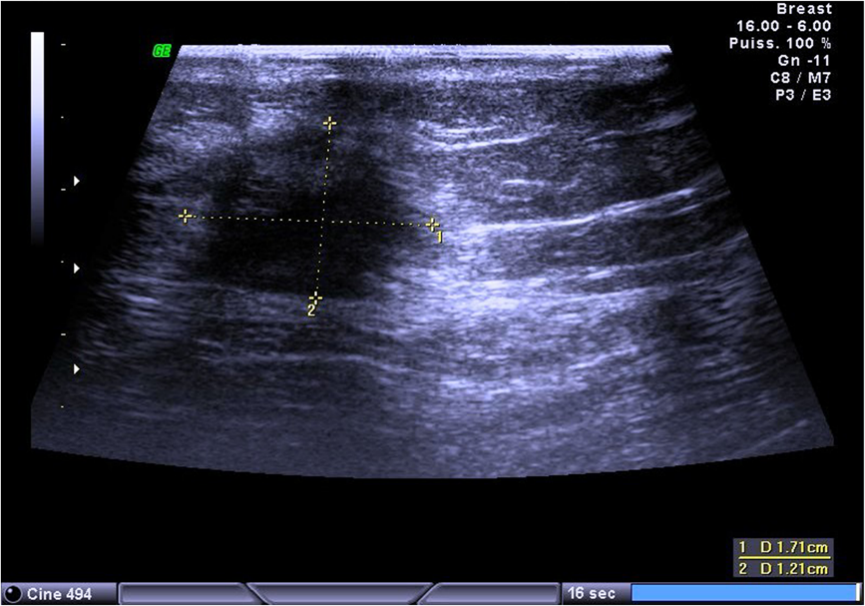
Ultrasound demonstrated a 17mm hypoechoic, heterogeneous, irregular and poorly limited mass, suspicious for malignancy.
